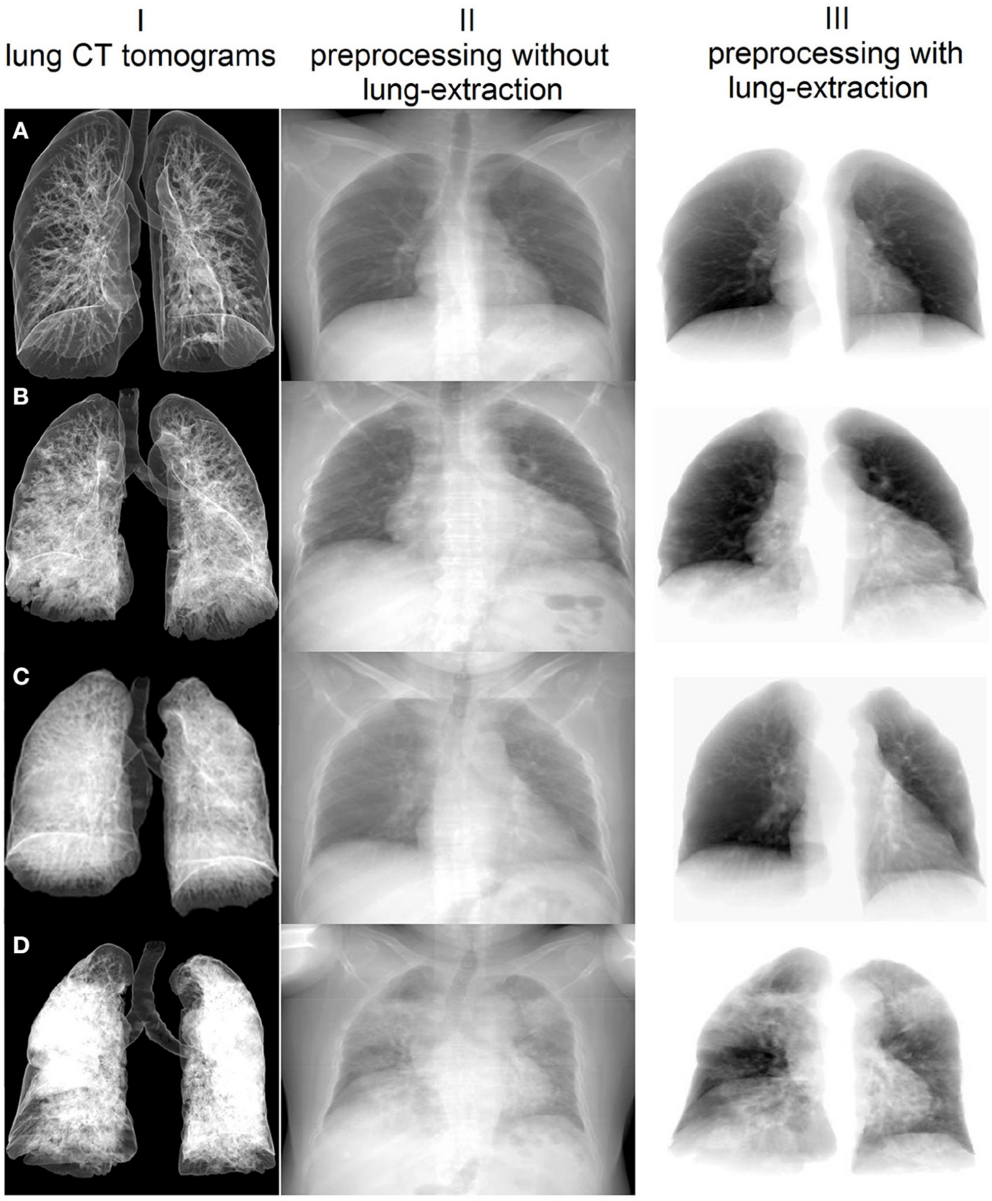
Sample images extracted with the proposed pre-processing steps and averaged over the coronal plane of the lung CT examination. I – Lung CT presented with volume rendering technique; different percentages of lung involvement: (A) 1.16%, (B) 15.83%, (C) 29.09%, (D) 62.29%. Pre-processed 2D images with (II) and without background (III).
